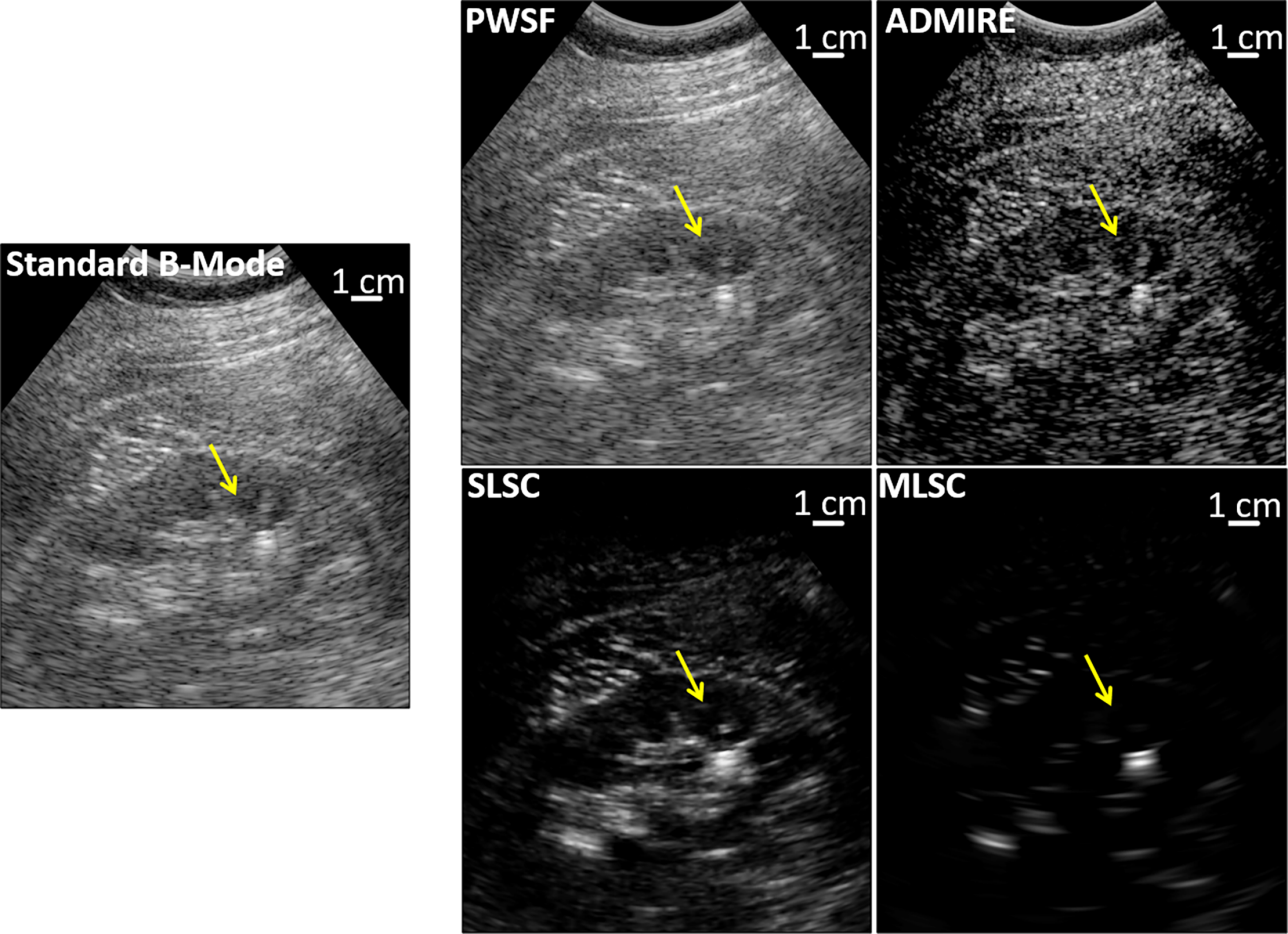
In vivo kidney stone case with standard B-mode and under the advanced beamforming methods. This patient had a CT scan showing a 14cm skin-to-stone distance, which is notable since depths >10cm are generally considered challenging with clinical ultrasound. PWSF is similar to B-mode except transmit focusing is performed everywhere in the image. Under ADMIRE, SLSC, and MLSC, the stone (yellow arrow) appears more echogenic. MLSC also suppresses the signal from the surrounding tissue.
